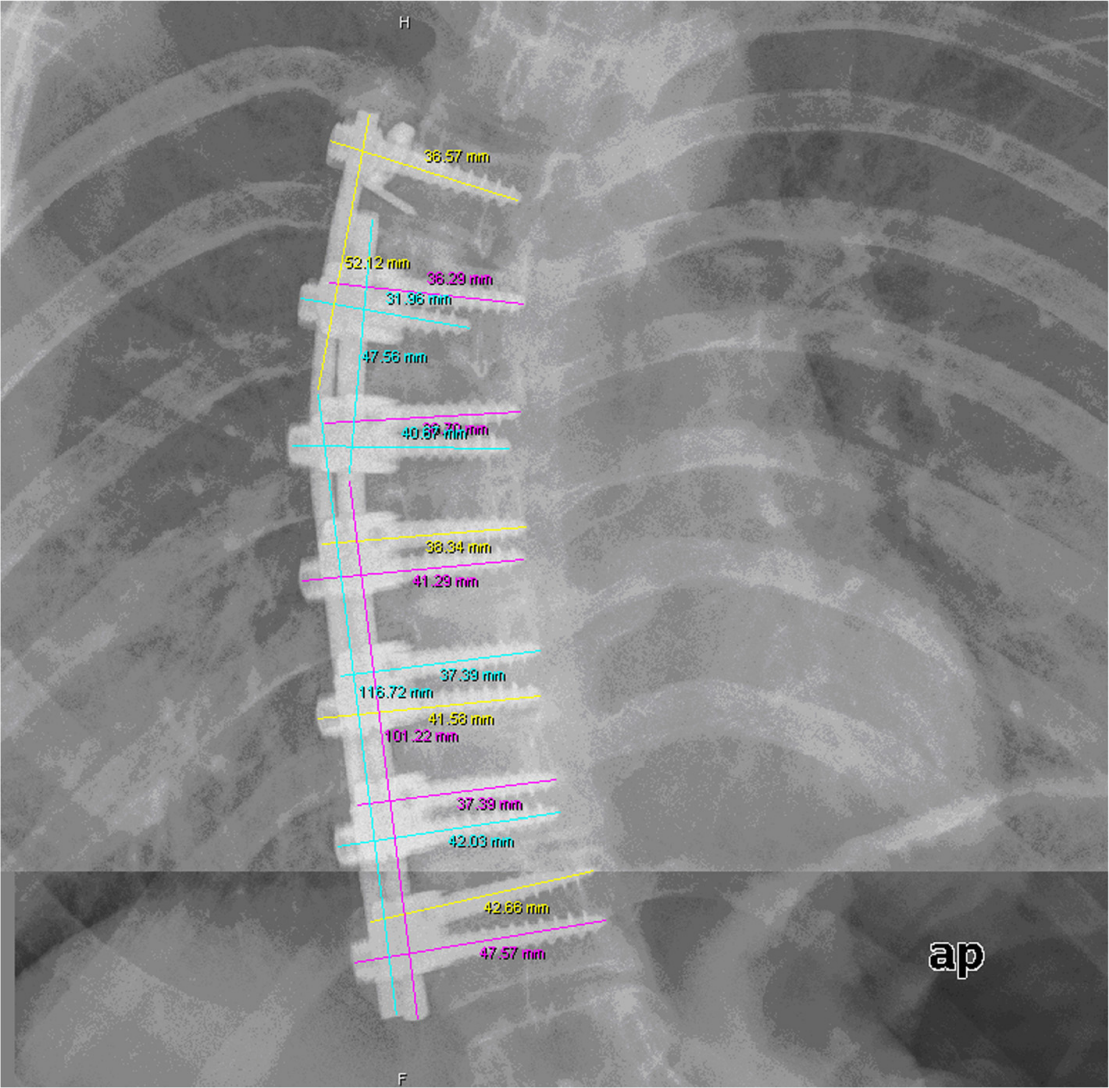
Technique of length-measurement of the spinal fusion on lateral radiographs of the spine. The length of each screw and rod were added to obtain the total length of spinal fusion.
